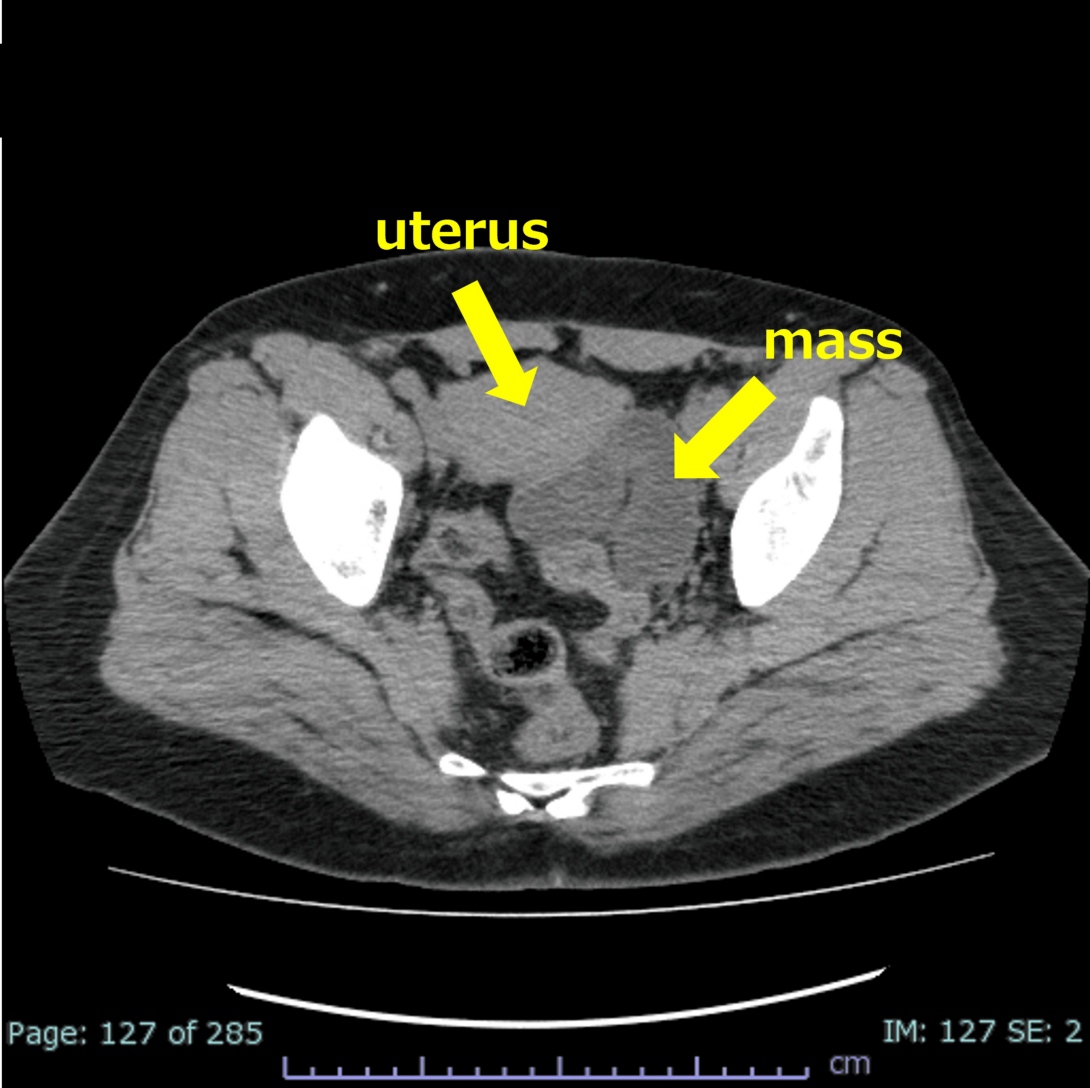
CT scan findings. The patient's CT scan showed a sausage-shaped enlarged mass on the left posterior of her uterus, which was suspected to be a torsion of the fallopian tubes based on the imaging results.
